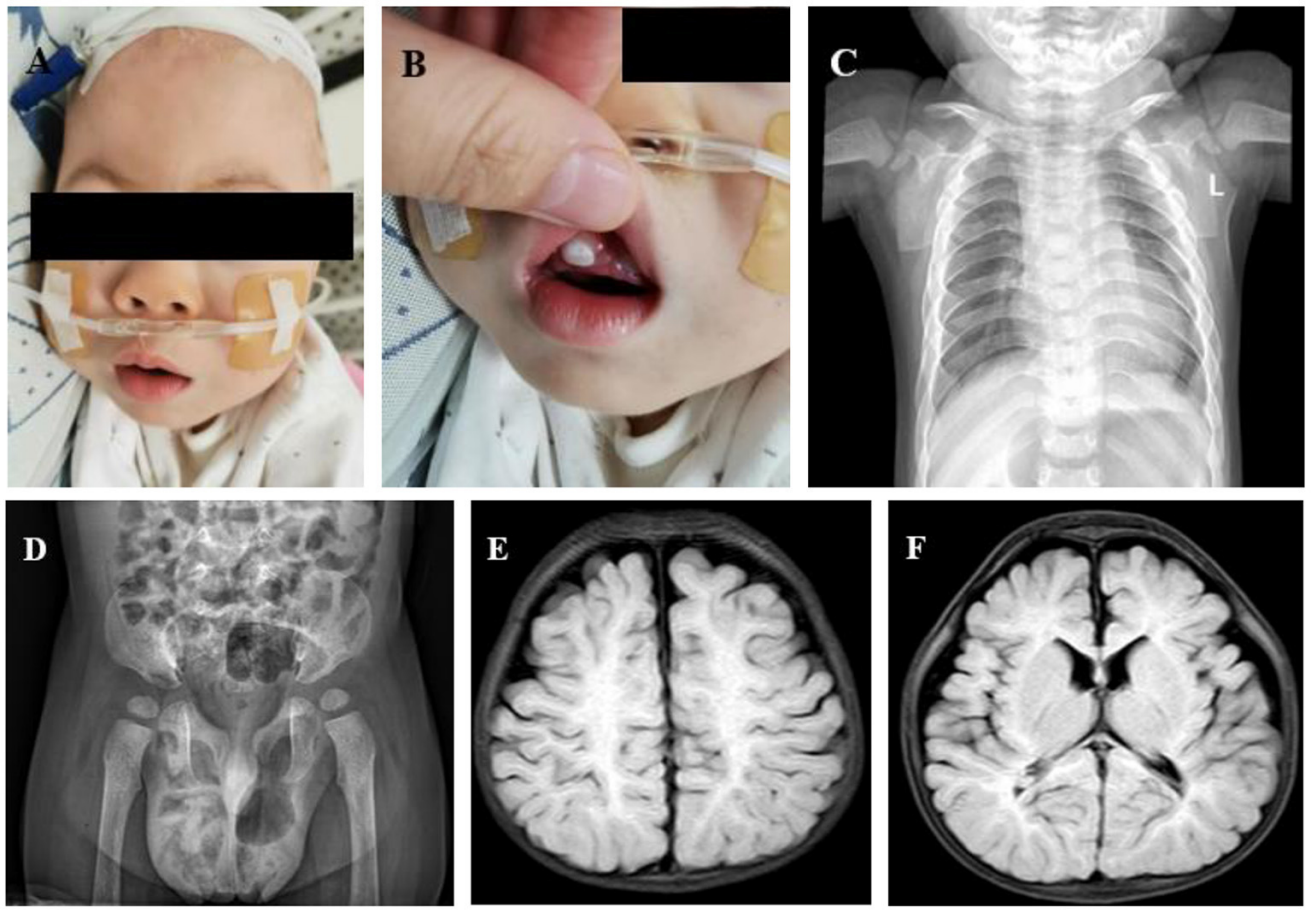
(A) Abnormal facial features (thick arched eyebrows, a flat nose, swollen eyelids, exophthalmos, epicanthus, low-set ears, and a short neck). (B) Obvious gingival hyperplasia. (C) Anteroposterior chest X-ray: small chest shape, short floating ribs, and irregular bilateral proximal humerus. (D) Pelvis anteroposterior X-ray: sharp and deep bilateral sciatic incisions; small and shallow bilateral acetabulums. (E) Brain MRI: small bilateral frontotemporal lobes, wide extracerebral space, and less white matter. (F) Brain MRI: thinner corpus callosum.
